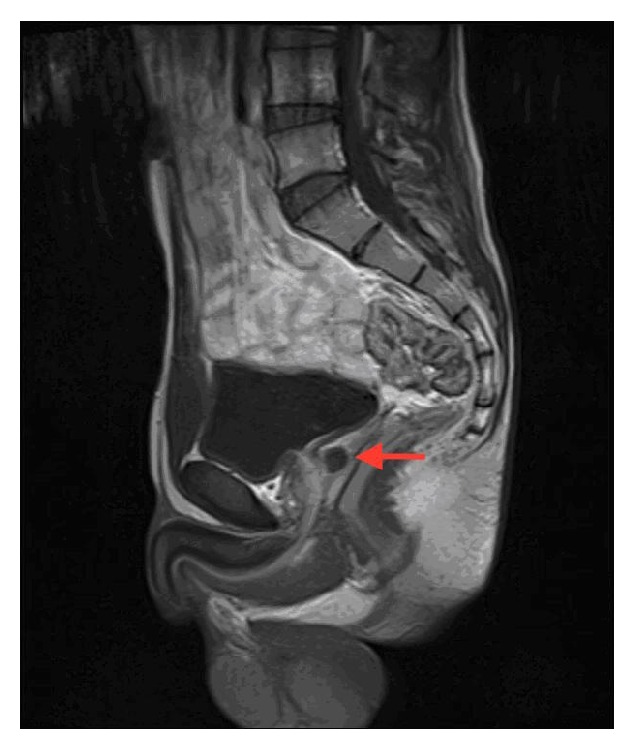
Prostatic midline cyst MRI.
